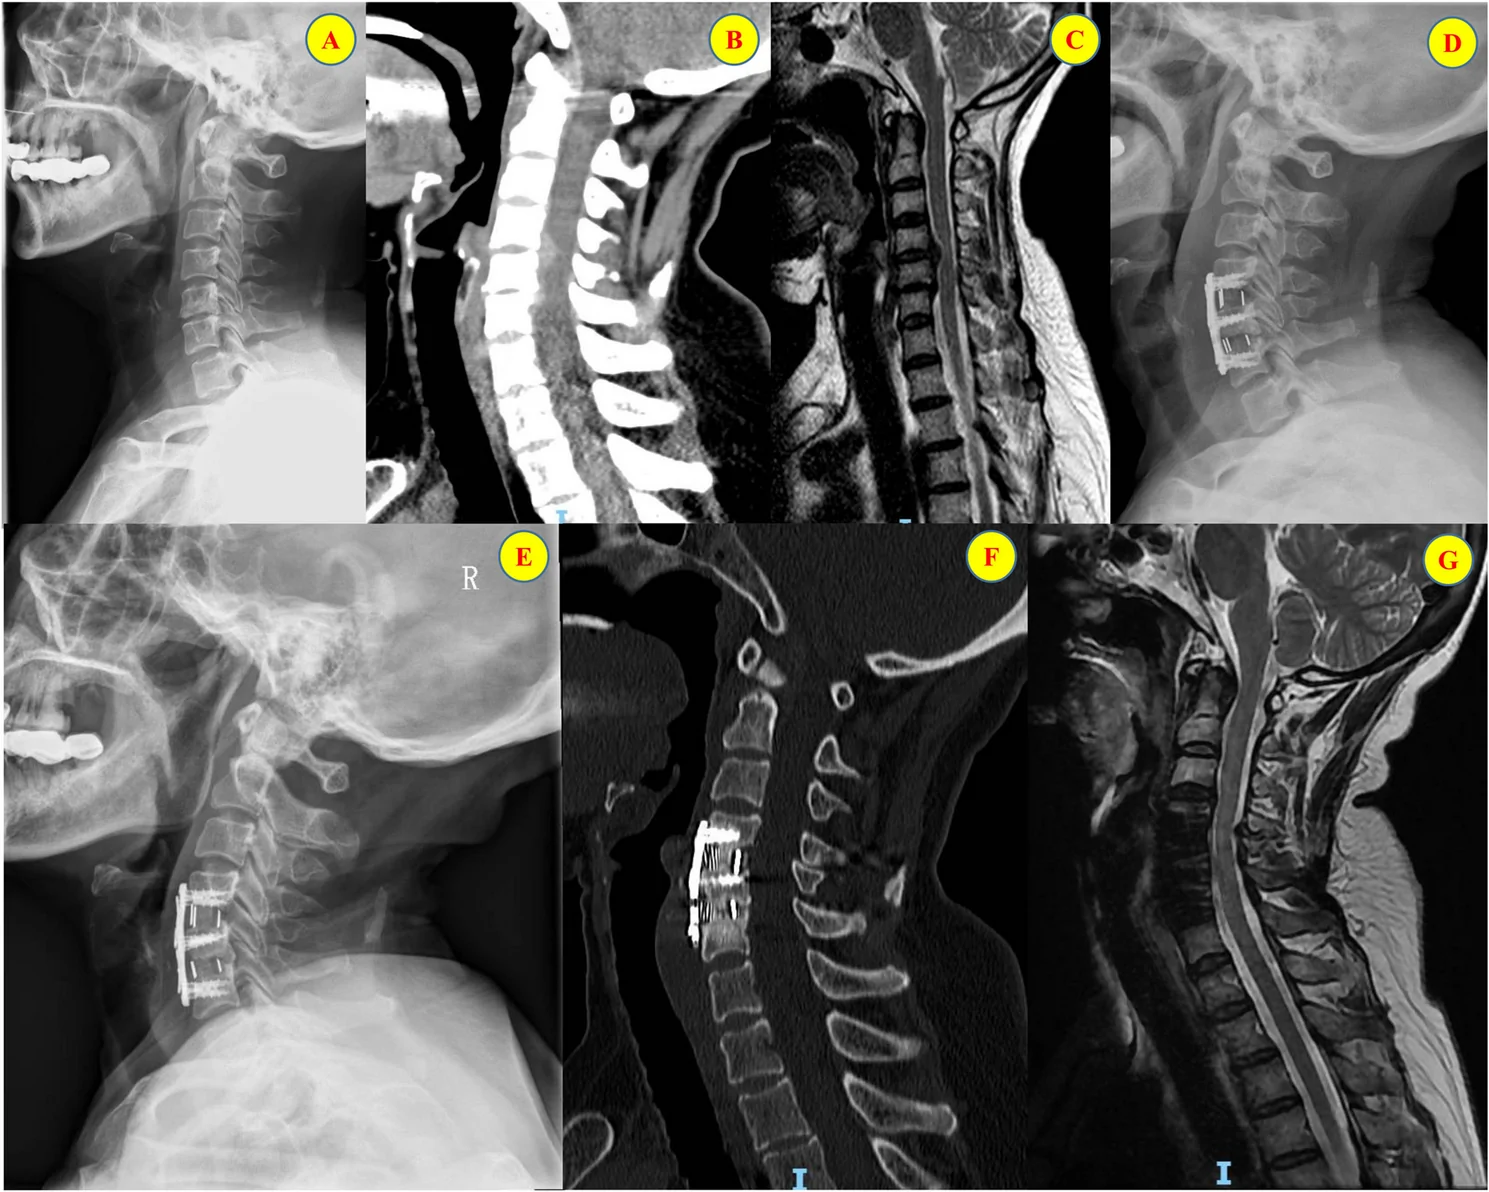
A 62-year-old male patient underwent ACDF for C4‒C6 segmental pathology. Preoperative imaging (A-C) revealed disc herniation at the C4/5 and C5/6 levels with corresponding segmental spinal canal stenosis, marked spinal cord compression at C4/5, and loss of cervical lordosis. Immediate postoperative radiographs (D) demonstrated good positioning of the internal fixation. Follow-up imaging at 2 years (E-G) further confirmed stable internal fixation placement, good restoration of the cervical sagittal alignment, and bony fusion at the operative level, indicating durable and stable surgical outcomes
